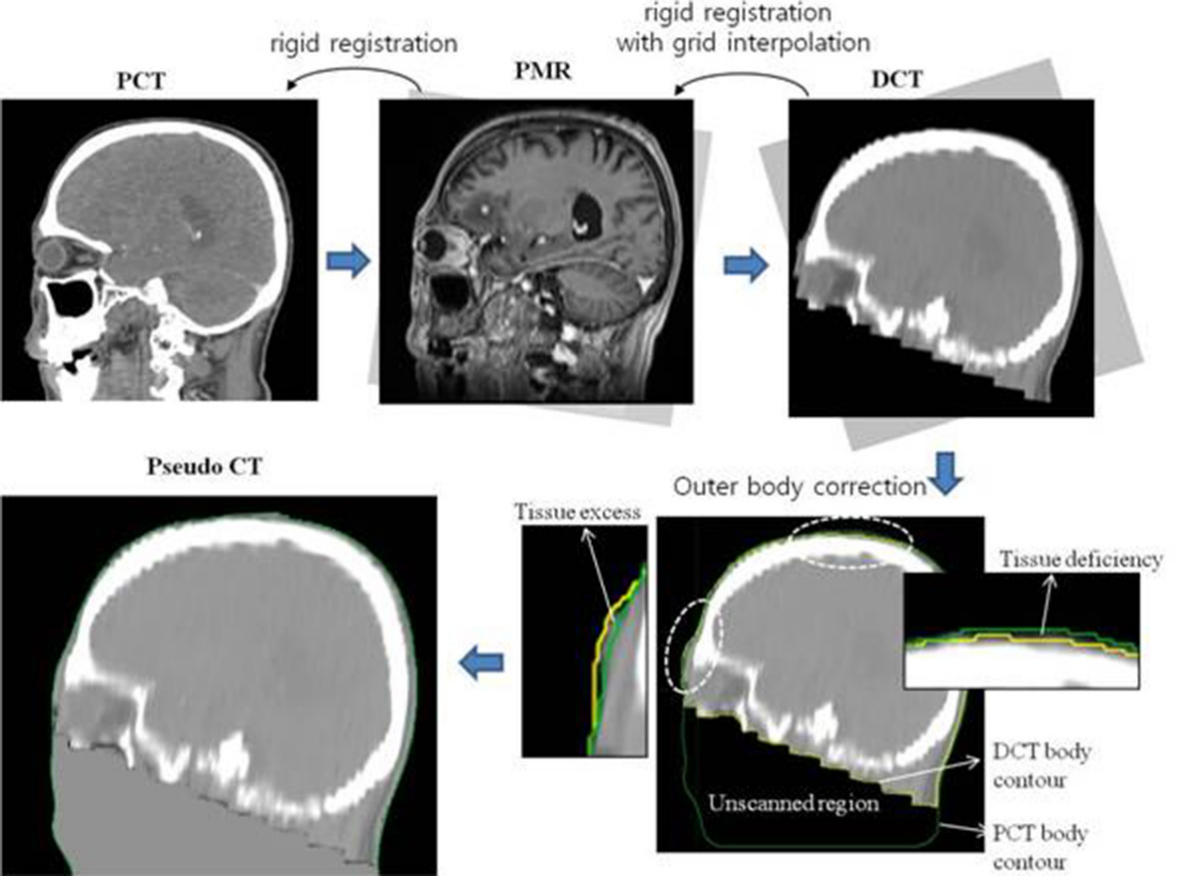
Work flow for pseudo-CT generation method used in the study
